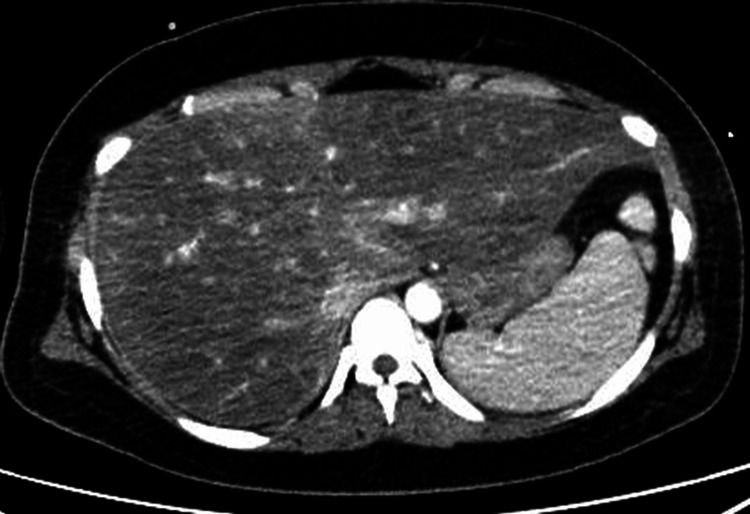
Axial abdominal contrast-enhanced CT image taken postoperatively shows marked fatty liver and hepatomegaly.
